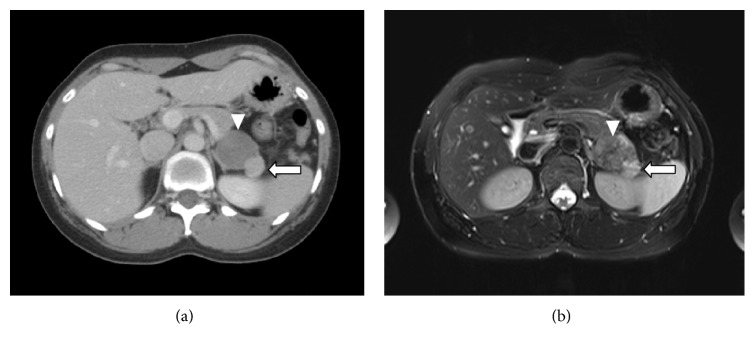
Imaging findings of the patient. (a) Computed tomography shows two adjacent round solid masses in the left suprarenal fossa: a 4 cm low-density mass (arrowhead) and another 1.8 cm enhancing mass (arrow). (b) On T2 magnetic resonance imaging, the 4 cm mass (arrowhead) showed intermediate signal intensity with subtle peripheral and delayed centripetal enhancement in the arterial phase, suggesting a neurogenic tumor. In contrast, the 1.8 cm mass (arrow) showed similar intensity to the spleen.
